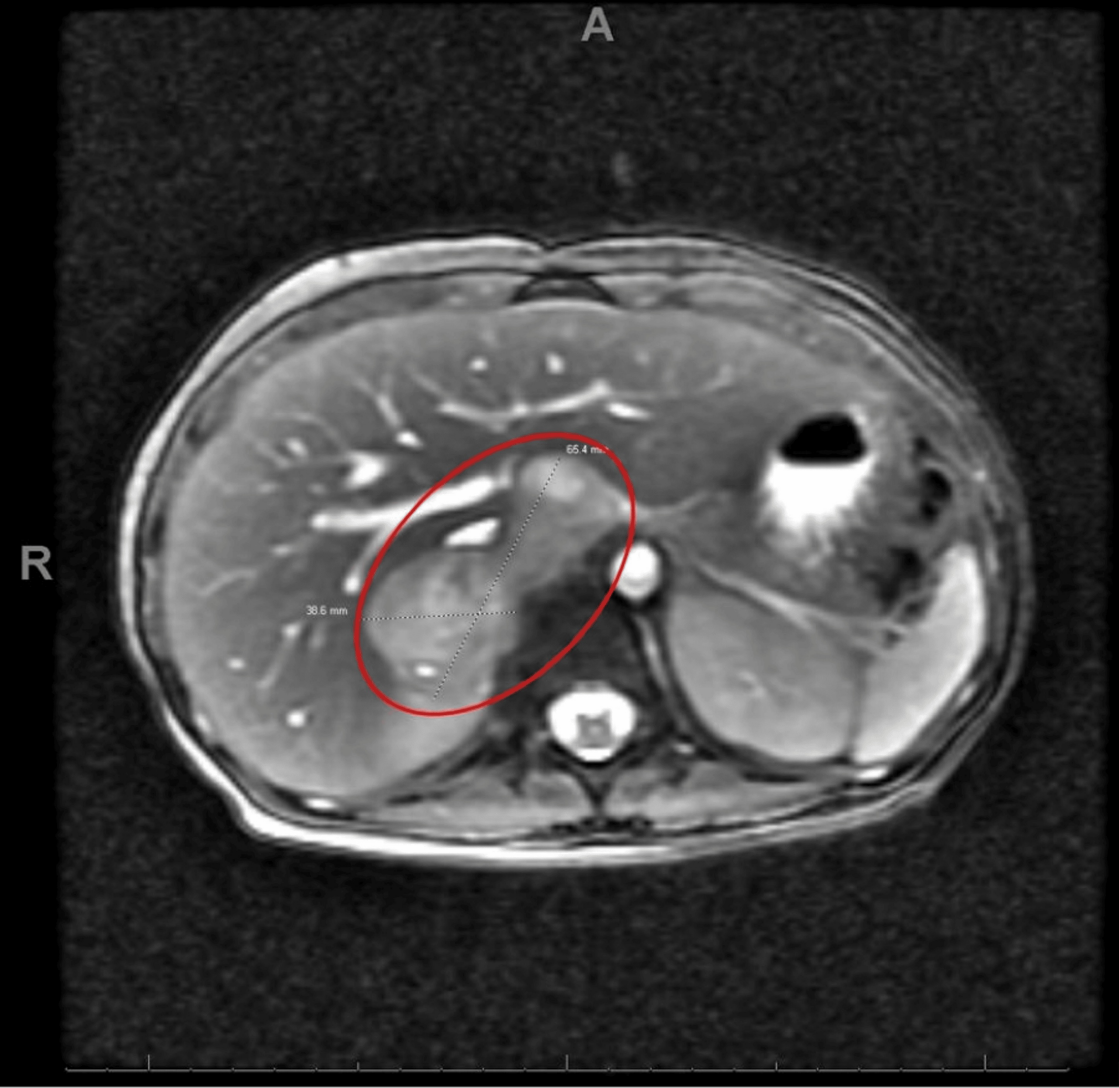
Axial plane MRI view highlighting the suspected pheochromocytoma. The mass is highlighted by a red circle.
